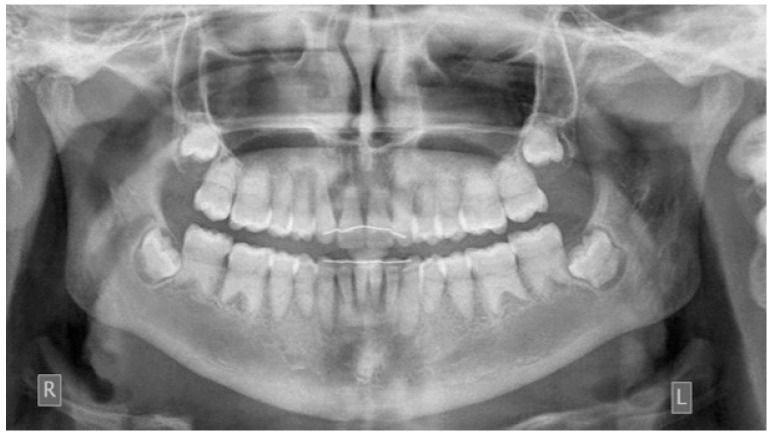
Post-treatment panoramic radiograph. No root resorption was observed (R: right, L: left).
